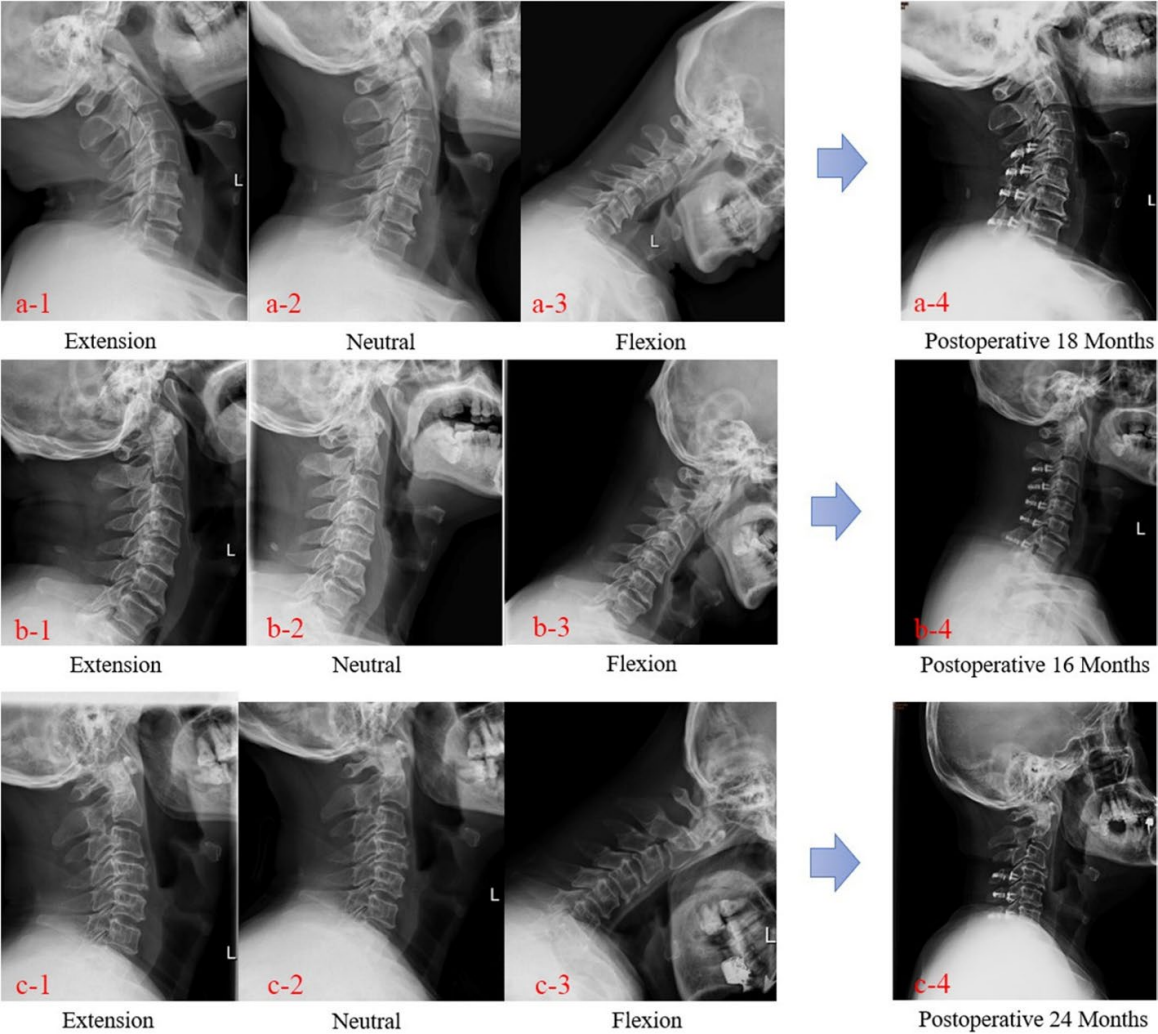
Representative cases. Sagittal preoperative radiographs (a-1, 2, 3) showing increased Ext ROM (20.3°) in a 65-year-old man who underwent laminoplasty of C4–7; no significant LCL was observed 18 months after surgery (a-2, a-4: pre CL 20.1° vs. post CL 24.9°). Sagittal preoperative radiographs (b-1, 2, 3) showing low Ext ROM (8.2°) and Flex ROM (29°) in a 48-year-old man who underwent laminoplasty of C3–7; mild LCL was observed 16 months after surgery (b-2, b-4: pre CL 16.2° vs. post CL 8.6°). Sagittal preoperative radiographs (c-1, 2, 3) showing low Ext ROM (3.1°) and increased Flex ROM (38.3°) in a 55-year-old man who underwent laminoplasty of C4–6; severe LCL was observed 24 months after surgery (c-2, c-4: pre CL 15.4° vs. post CL -7.6°). CL, cervical lordosis; LCL, loss of cervical lordosis; Flex ROM, cervical spine range of flexion; Ext ROM, cervical spine range of extension
